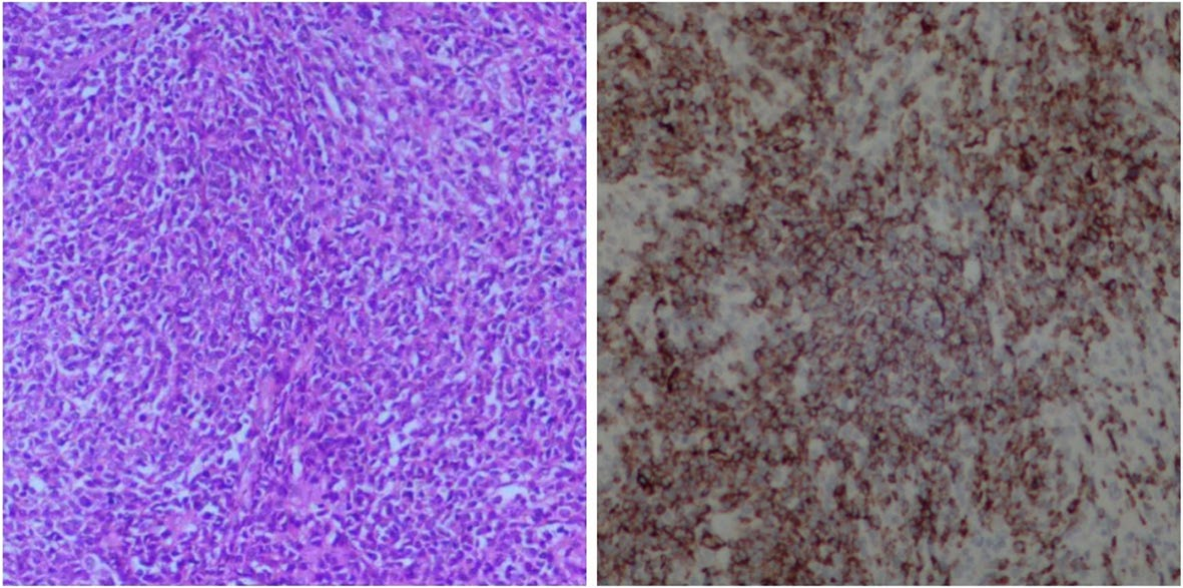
CT-guided pancreatic puncture suspected myeloid sarcoma
